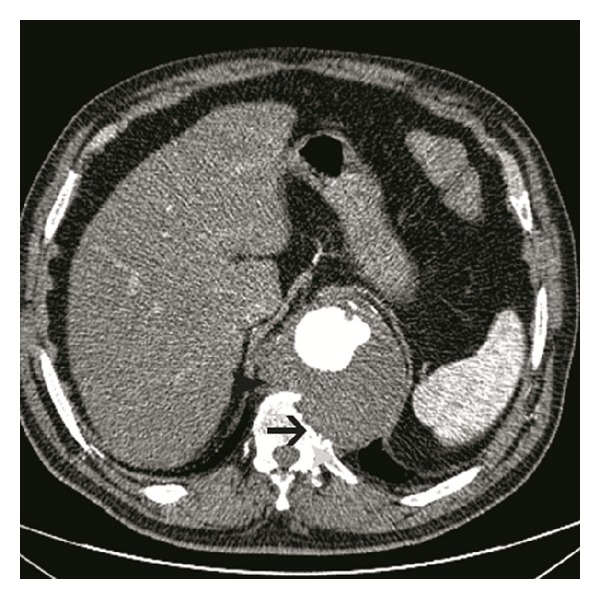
Contrast-enhanced axial multidetector CT angiography image obtained at the level of thoracal 11th vertebra reveals chronic-contained aneurysm rupture of the aorta with vertebral erosion and lysis. Draped aorta sign is present (arrow). Aortic lumen was irregular at the left side.
